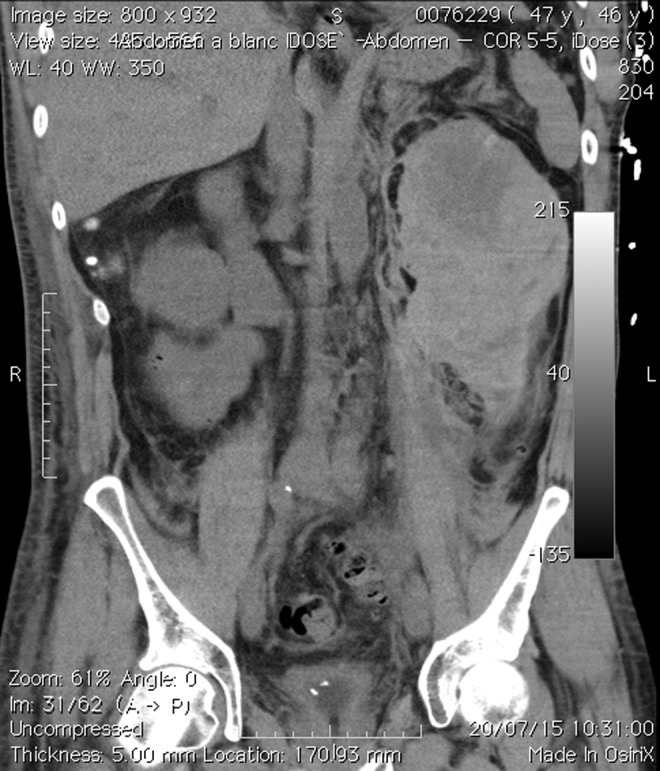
Coronal CT image of a massive subcapsular and retroperitoneal renal hematoma in the left hemiabdomen after a third consecutive SWL treatment of a calculus in the left UPJ. SWL, extracorporeal shockwave lithotripsy; UPJ, ureteropelvic junction.
